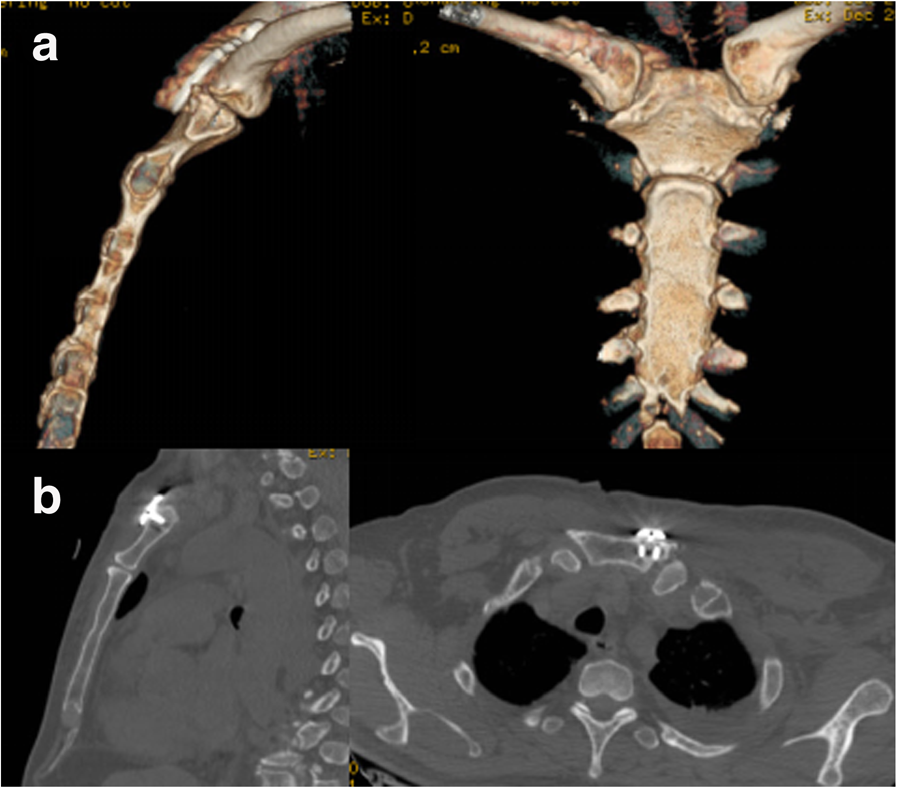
Postoperative A postoperative three-dimensional computed tomography scan a showing good reduction and fixation with the locking plate, sagittal and transverse computed tomography b scans showing unicortical screw fixation in the sternum
